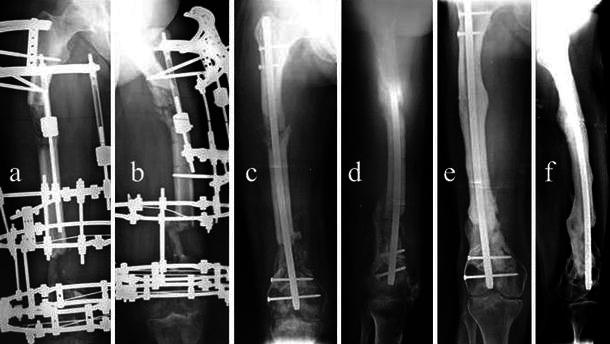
Case 3: a, b preoperative X-ray; bony bridge in the docking site, arrest of transport, varus, and procurvatus of the regenerated bone, c, d follow-up 40 days later, e, f follow-up 4 years later
